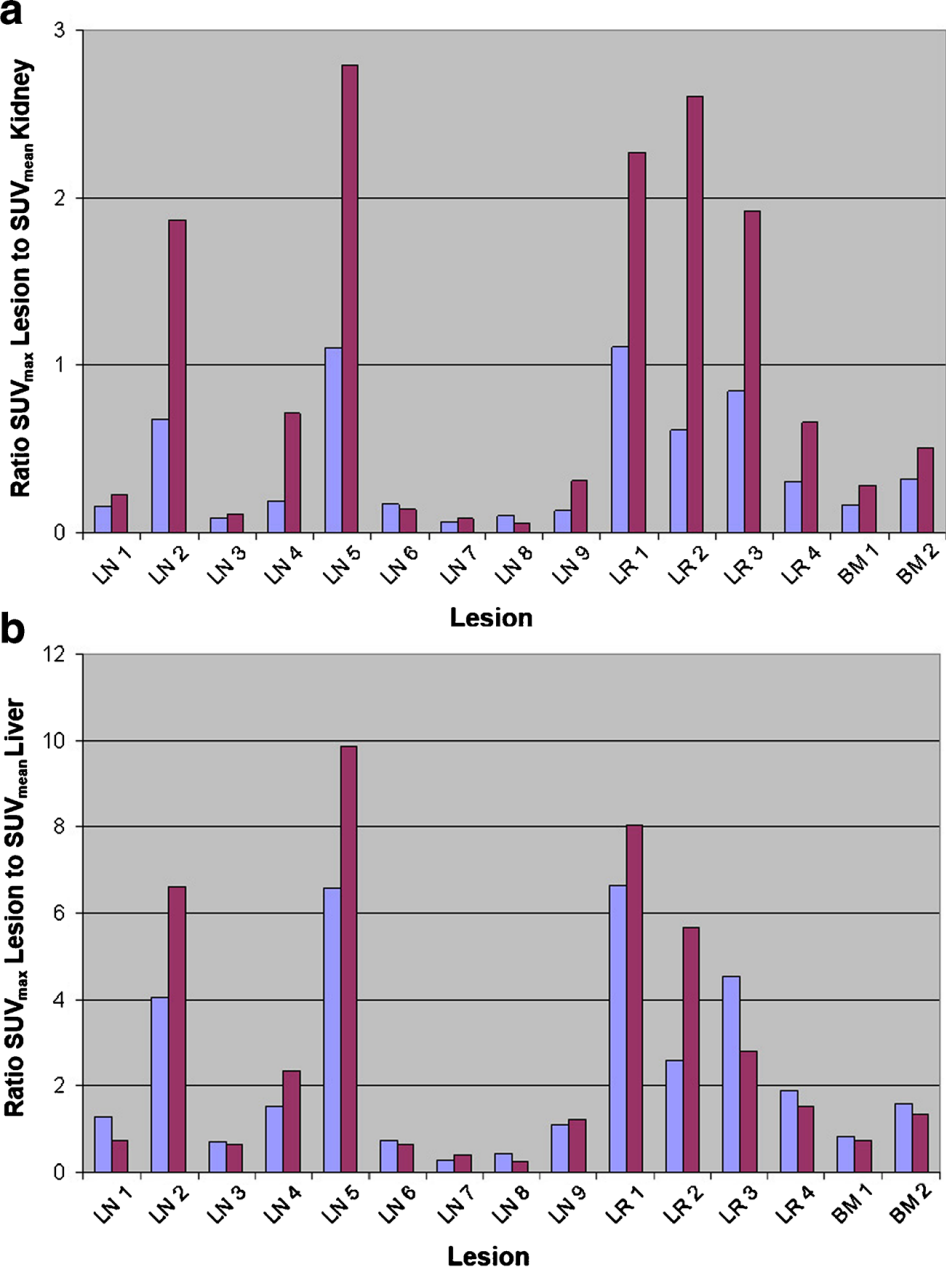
a Ratio of SUV_max_ in lesions to SUV_mean_ in the kidney and b ratio of SUV_max_ in lesions to SUV_mean_ in the liver. The *blue columns* represent [^68^Ga]Ga-PSMA-HBED-CC PET/CT, and the *red columns* represent [^18^F]DCFPyL PET/CT. [^18^F]DCFPyL showed a higher tumor to background ratio than [^68^Ga]Ga-PSMA-HBED-CC when the kidney was used as a reference organ (*p* = 0.006, *n* = 15). The tumor to background ratio did not differ significantly between [^18^F]DCFPyL and [^68^Ga]Ga-PSMA-HBED-CC when the liver was used as a reference organ (*p* = 0.167, *n* = 15). *LN* lymph node metastasis, *LR* local relapse, *BM* bone metastasis.
